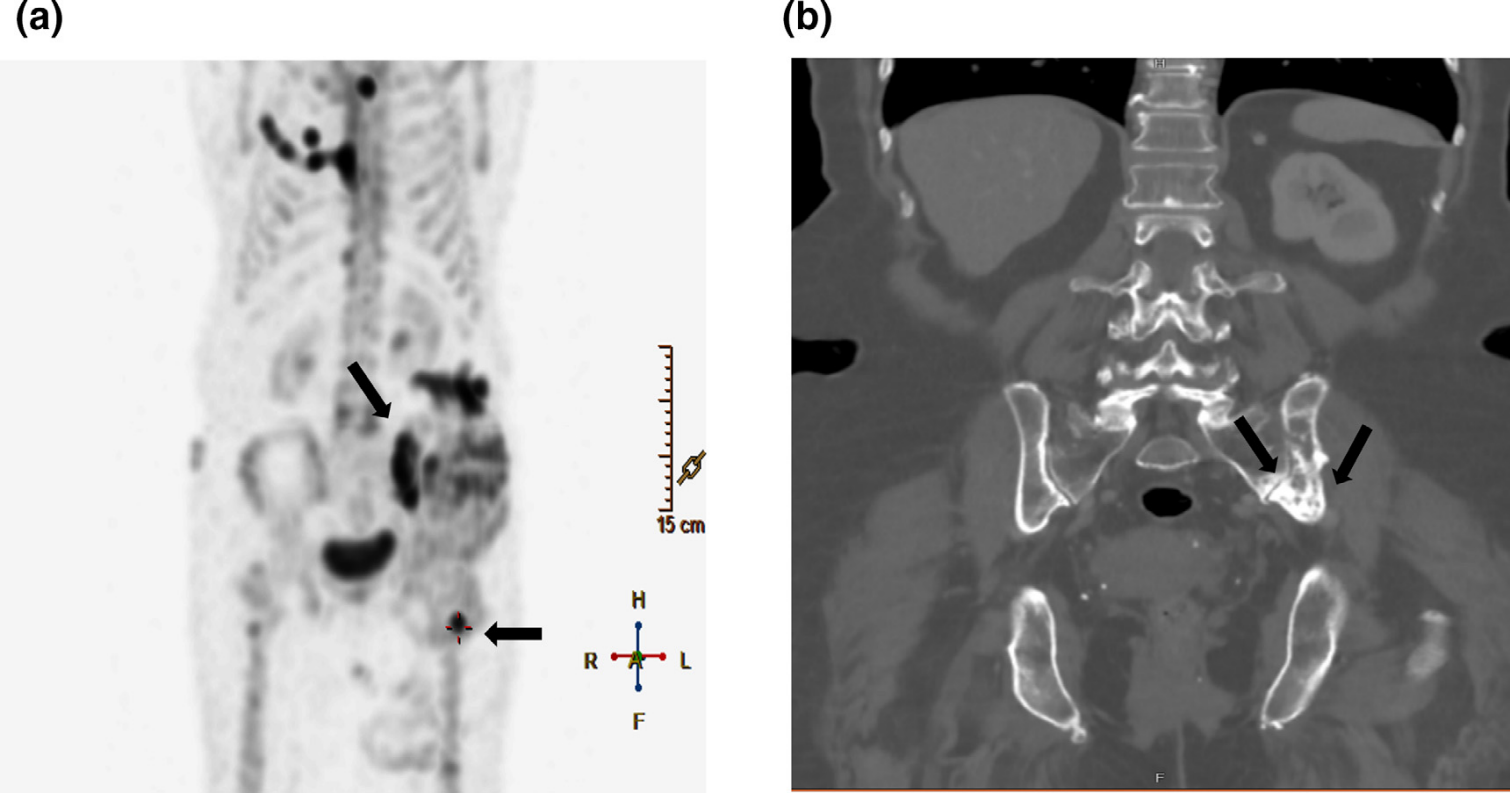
Bone scintigraphy (A) and computed tomography coronal view (B) showing the site of metastatic disease in bone involving left ilium and left femur (represented with arrows).
